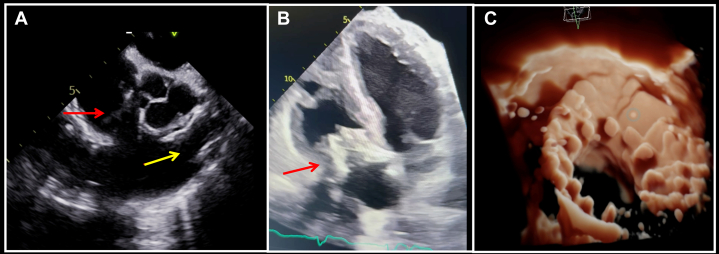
Periprocedural and Postprocedural Echocardiography (A) Transesophageal echocardiography mid-esophageal right ventricular inflow-outflow view showing the tricuspid bioprosthesis (red arrow) and pulmonary bioprosthesis (yellow arrow). (B) Transthoracic apical 4-chamber view showing the tricuspid bioprosthesis (red arrow). (C) Three-dimensional reconstruction of the tricuspid bioprosthesis.
